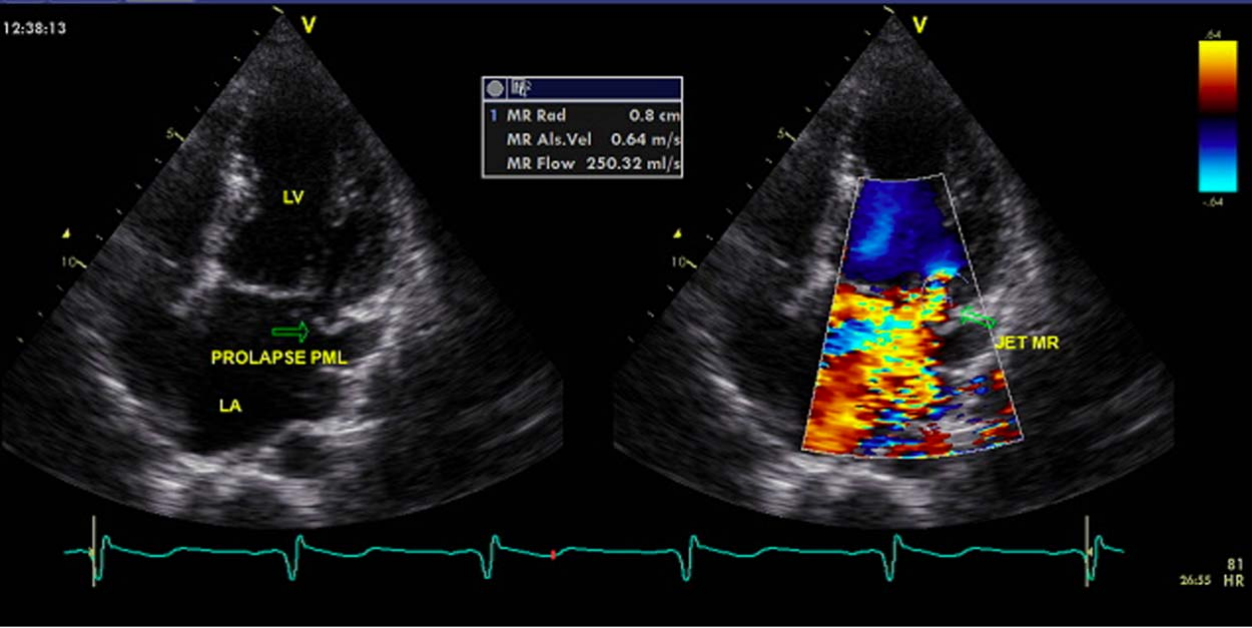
Patient 1 echocardiography. Echocardiography showed left ventricular dilatation, preserved ejection fraction, and severe mitral regurgitation with PML prolapse.
